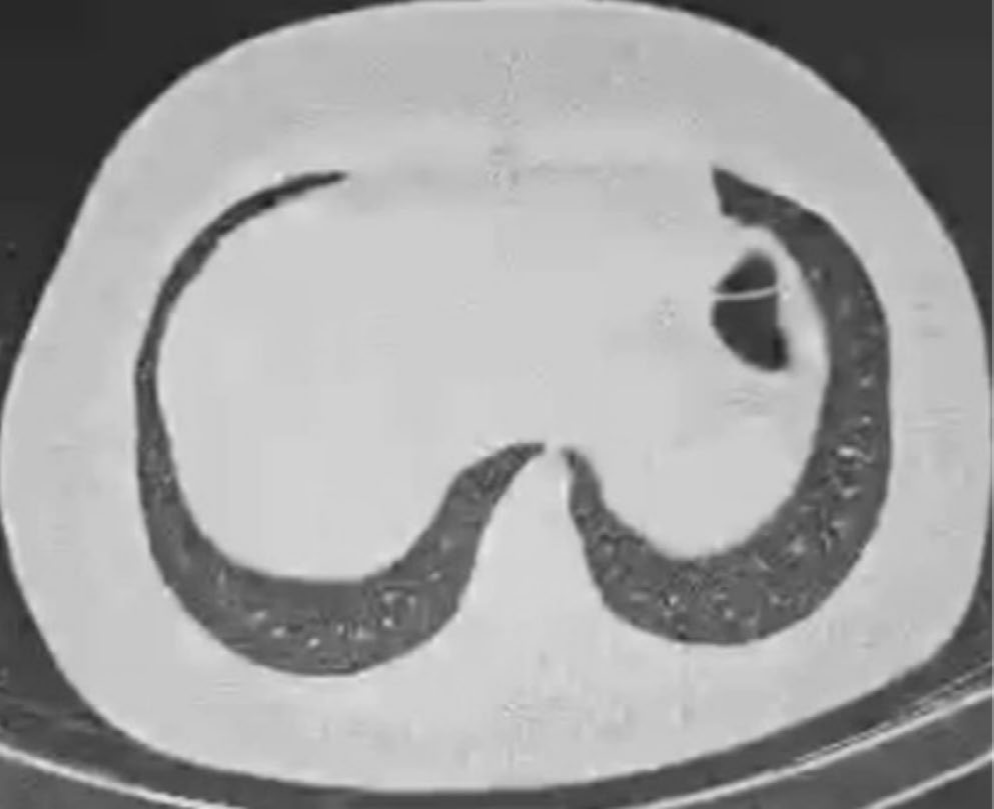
CT scan of the chest after 6 months of treatment showing complete resolution of liver abscesses and pleural effusion.
